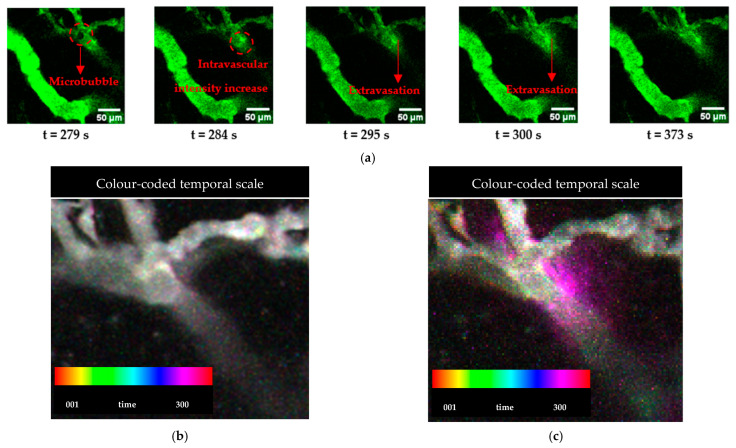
Time series of real-time MP-IVM during ACT^®^ showing a microbubble lodged at a branching point and dextran extravasation (a). Colour-coded temporal representation of a zoomed-in view of the extravasation area, showing the temporal behaviour of the dextran before ACT^®^ (b) and extravasation during treatment (c). Time 0 is the onset of ultrasound.
